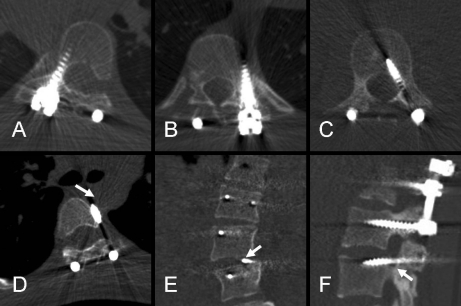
Axial (A–D), coronal (E) and sagittal (F) images obtained by low-dose spine CT from 6 patients. A. Normally-placed screw through the right pedicle of T7. B. Screw with total lateral cortical perforation (LCP grade 2) at the level of T9. C. Screw with total medial cortical perforation (MCP grade 2) at the level of L3. D. Pedicle screw with anterior cortical perforation (ACP) with screw tip in close proximity to the posterior-lateral limit of aorta (arrow). This screw was removed a few months later. E. Screw tip perforating the upper endplate of L4 (arrow) on the left side (EPP). F. Screw passing through the lower boundary of the neural foramen (FP) below L3 (arrow).
